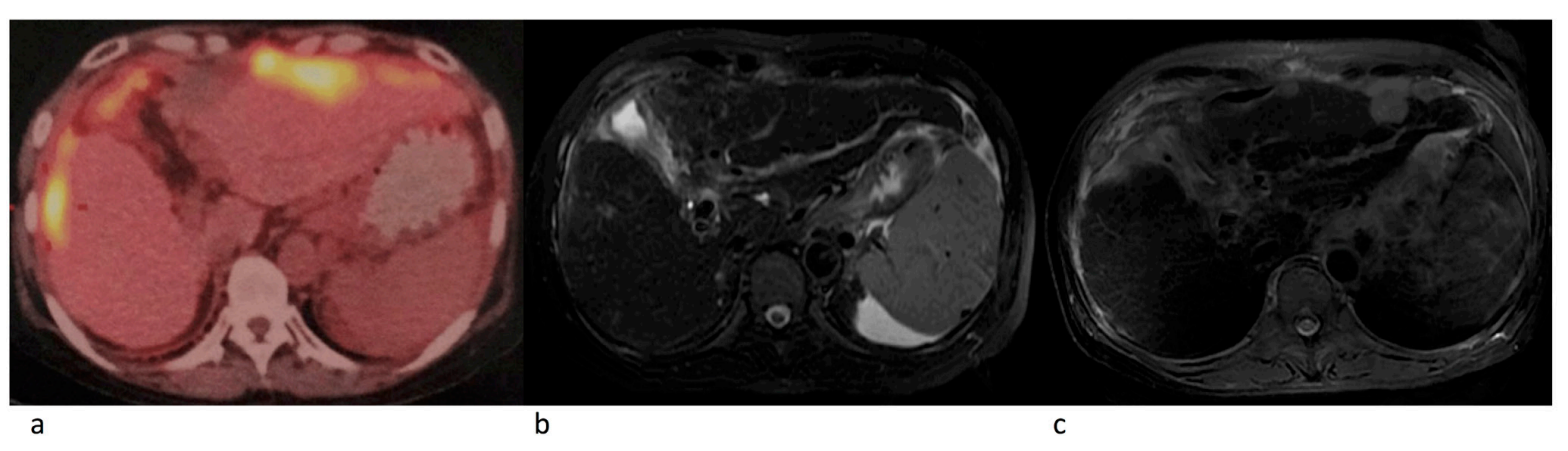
Radiographies of a 54-year old female patient PET-CT showed multiple lesions in bilobar, MRI (T2, after 7 cycles with nivolumab) showed lesions significant decreased. MRI (T2, two months after nivolumab was discontinued) showed progressive disease. a. Before nivolumab treatment. b. Seven cycles after nivolumab treatment. c. Two months later after nivolumab withdrawal.
